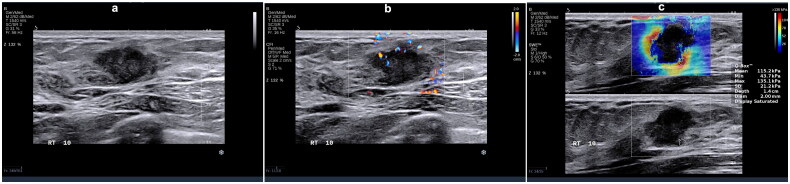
A 26-year-old female with right breast invasive ductal carcinoma grade III (score of 8), tumor size 2.0 × 1.5 × 1.0 cm, luminal B (HER2−) subtype. (a) Ultrasonography features: irregular shape, angular margins, no change in posterior echo, no calcification. (b) Adler blood flow grading – grade 1. (c) Emax = 135.1 Kpa.
